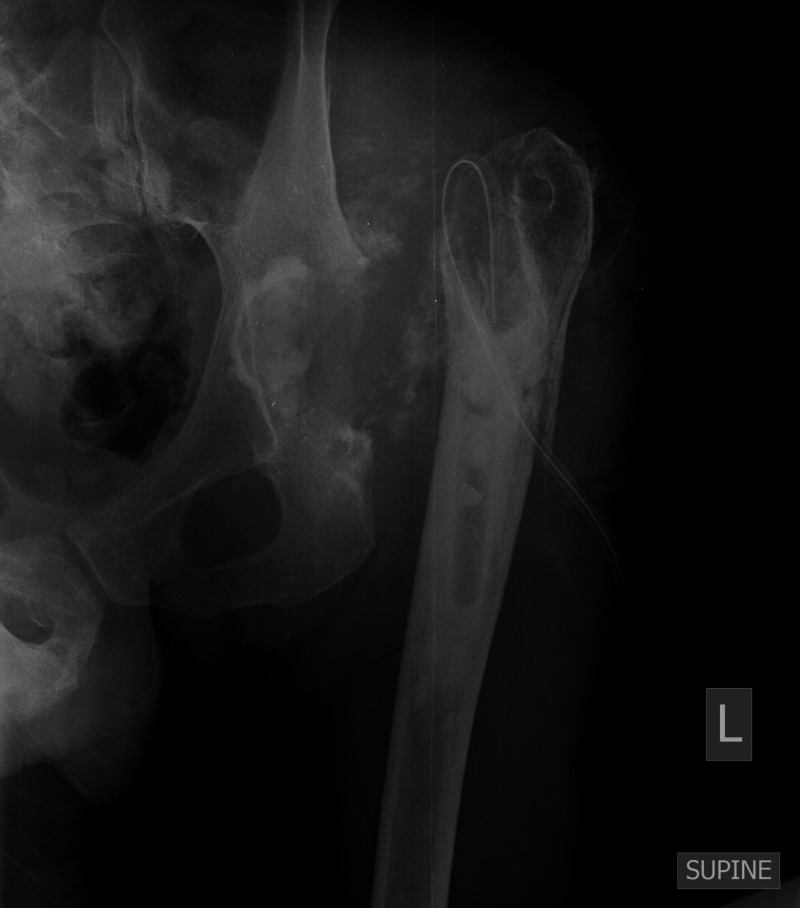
Postoperative anteroposterior left hip radiograph showing the resection Girdlestone procedure, removal of all implants and the acetabular cement
